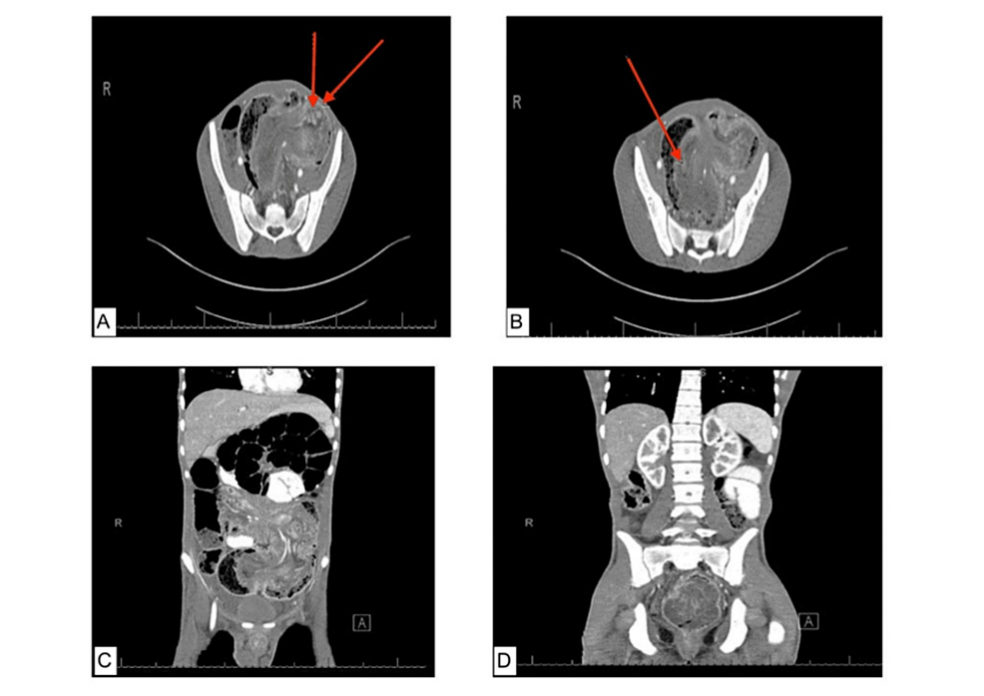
Computer tomography (CT) imaging A, B) Axial cuts of CT abdomen-pelvis with triple contrast (IV-oral-rectal) and the arrow pointing to the area of colo-colonic intussusception. C, D) Coronal cuts of CT abdomen-pelvis with triple contrast (IV-oral-rectal) with the intussusceptum reaching down to the rectum with mild subsequent small bowel dilatation.
